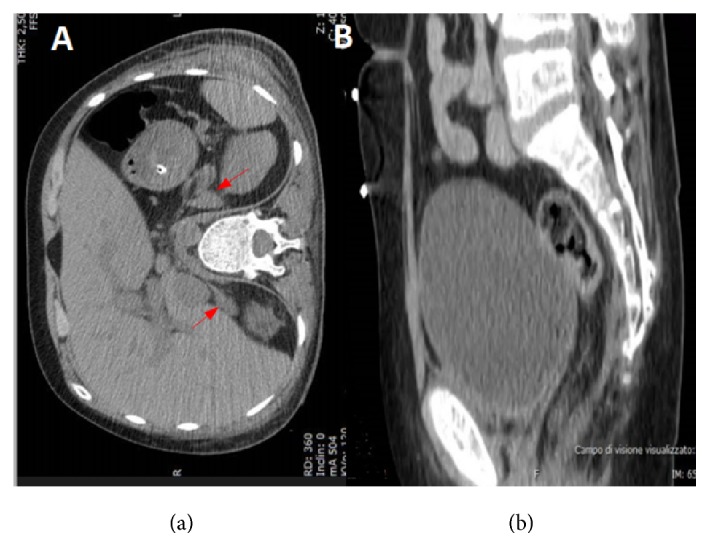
Abdomen and pelvis CT scan. (a) The adrenal glands are enlarged bilaterally (red arrow); (b) gonads are not detectable.
